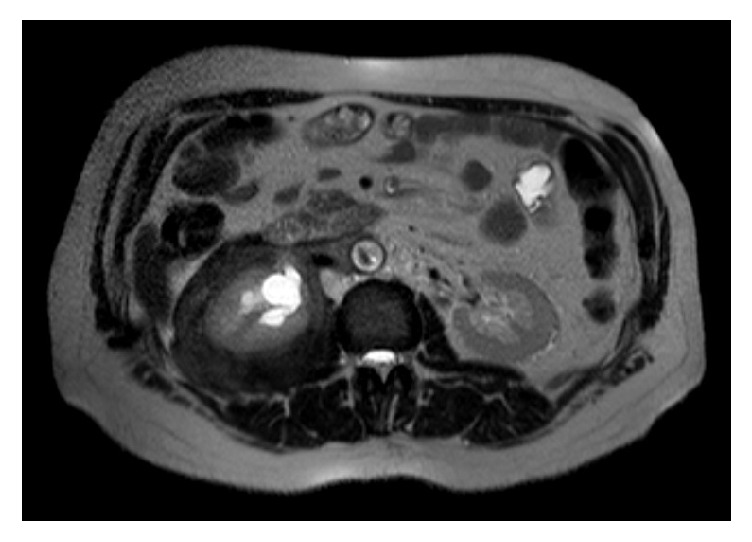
Preoperative magnetic resonance imaging (MRI) scan showing a right renal mass.
